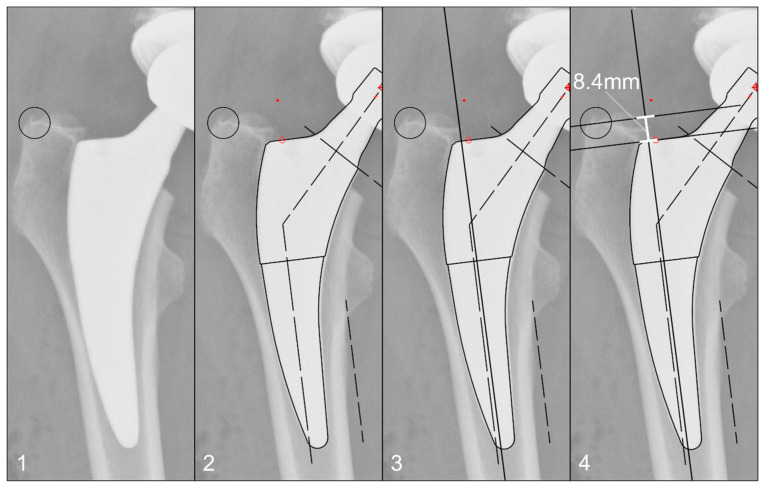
Method for measuring the stem position: (1) Identification of a clearly definable point on the greater trochanter, which appears identically on all radiographs up to 10 years postoperatively. (2) Calibration of the image using a planning template, as shown in Figure 2. (3) Determination of the femoral longitudinal axis. (4) Distance measurement of the defined point to the prosthetic shoulder along the femoral longitudinal axis.
